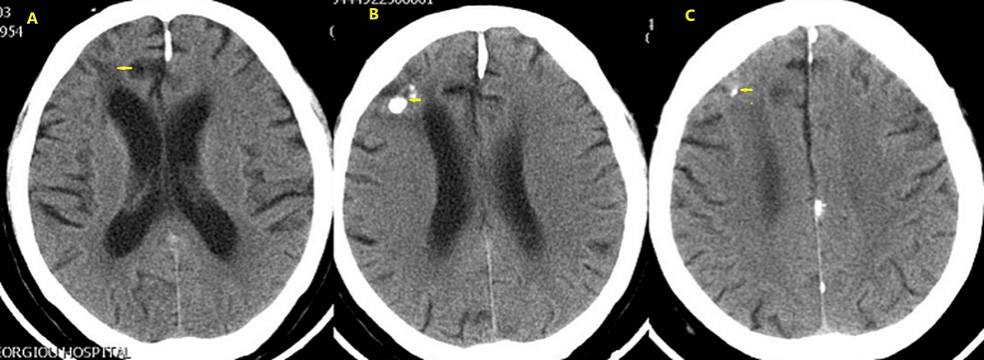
Brain computed tomography scan on January 7, 2011. Post-contrast axial images revealing a low-density area in front of the right frontal horn (A) and calcification in the right frontal lobe with no change in lesion dimensions and no enhancement (B, C). No other focal lesions are revealed.
